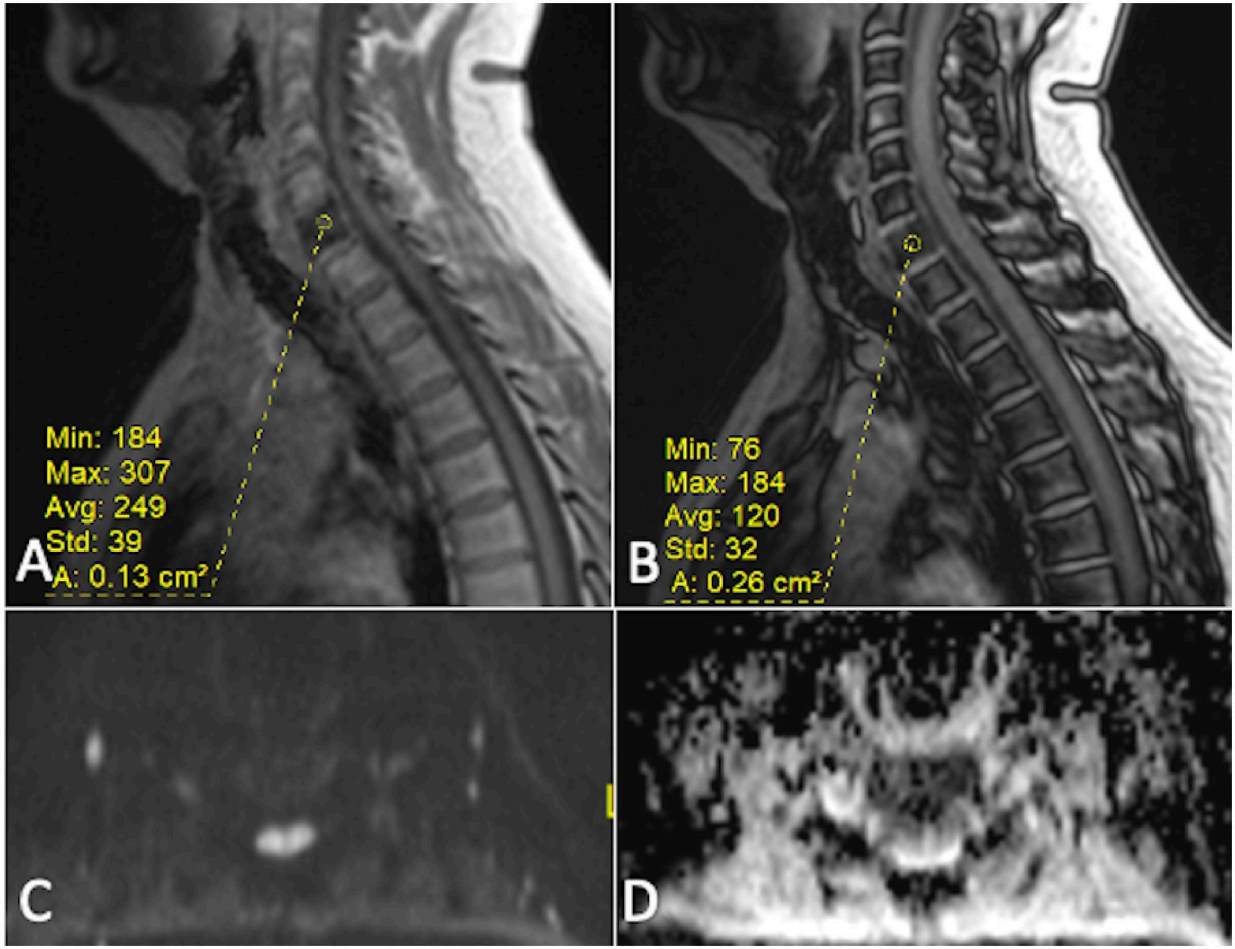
A focal area of red marrow hyperplasia in the C7 vertebra exhibits a 51.8% drop in signal on chemical shift imaging (A and B). This area shows no signs of diffusion restriction (C and D). More than 50% drop in signal intensity on out-of-phase chemical shift MRI images of the vertebral body, compared to in-phase images, indicates a benign lesion or normal bone marrow containing both fat and water, which cancel each other’s signals on the out-of-phase images.
